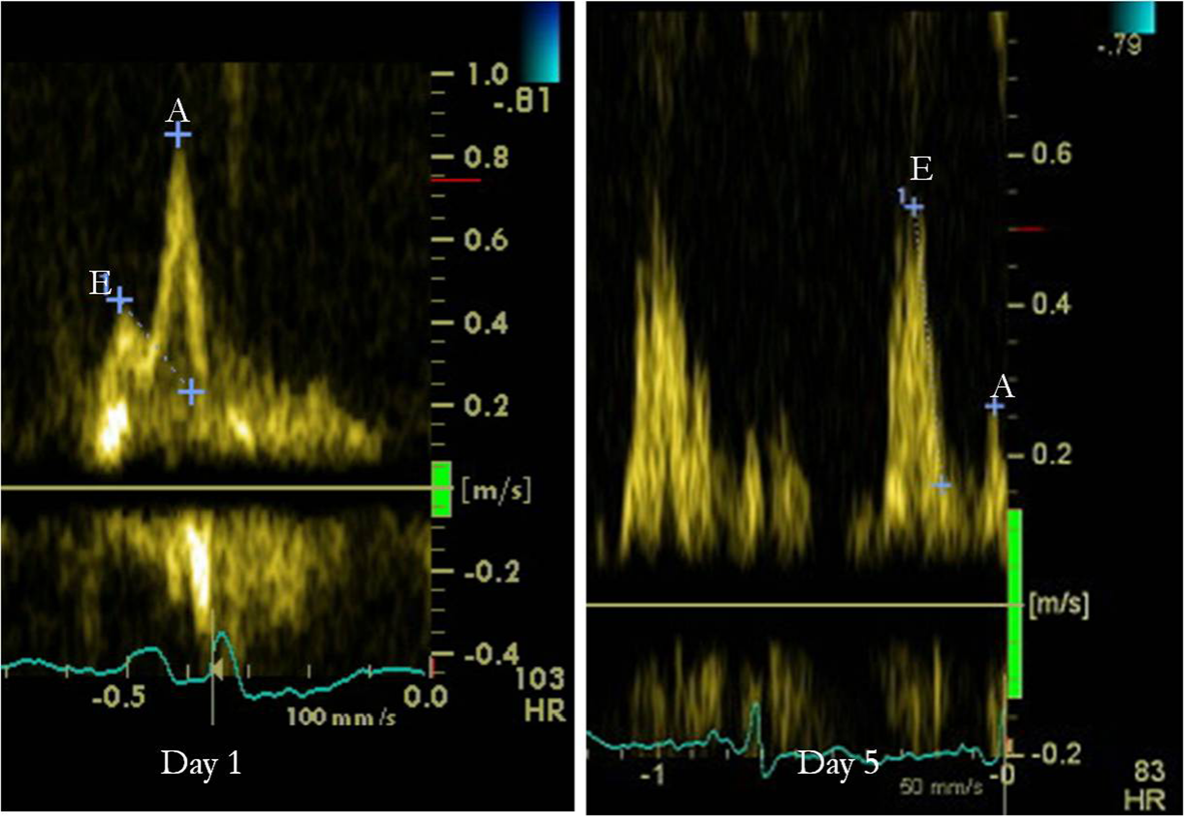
Echocardiogram mitral inflow pulsed wave Doppler showing reversal of E/A ratio indicating a restrictive pattern of filling at day 5.
